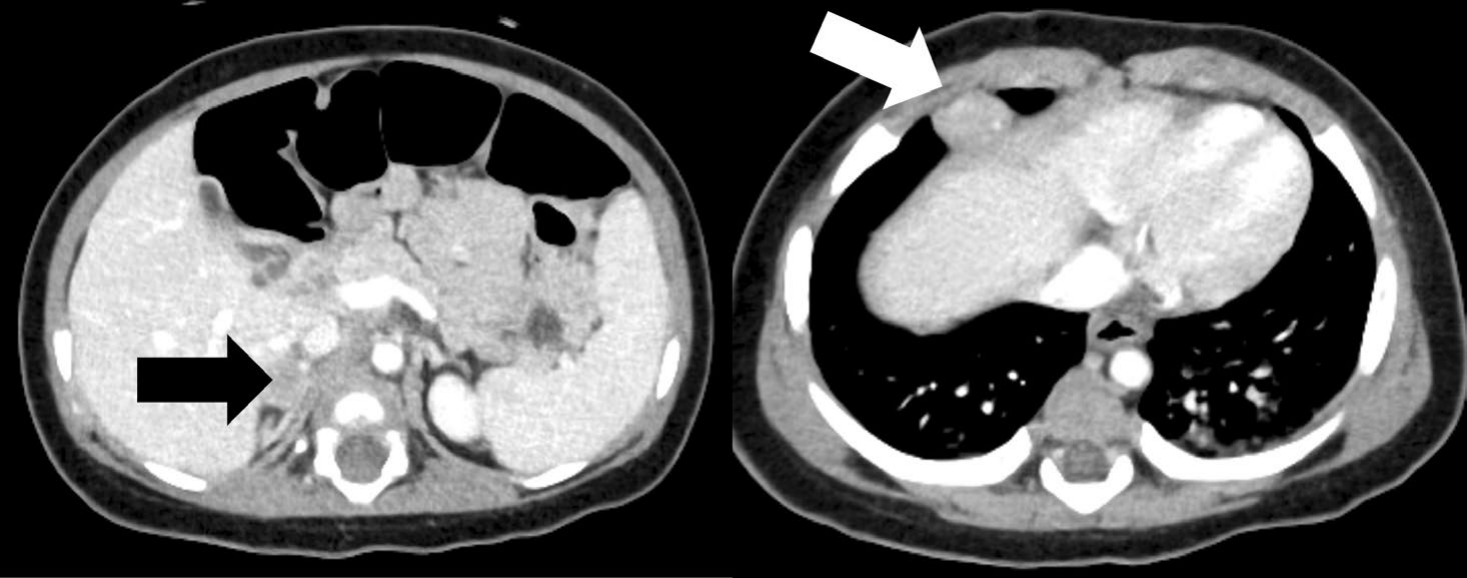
Computed tomography images at tumor progression. Left panel: The black arrow indicates the right adrenal mass. Right panel: The white arrow indicates the mediastinal lymph node.
